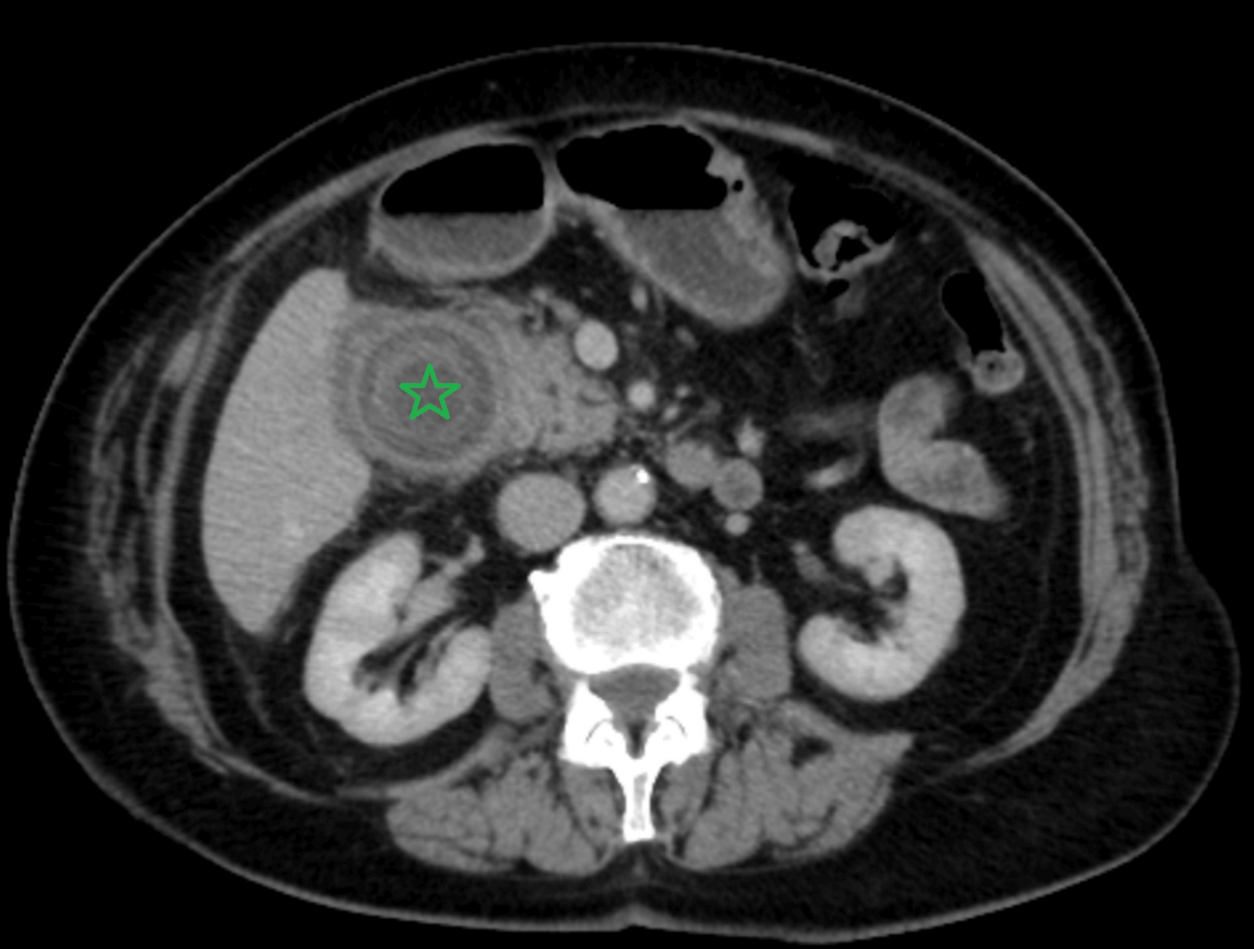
Abdominal CT scan three years ago: axial view showing a 31mm calculus present in the gallbladder neck with chronic cholecystitis. No evidence of a cholecysto-enteric fistula at this stage (green star)
